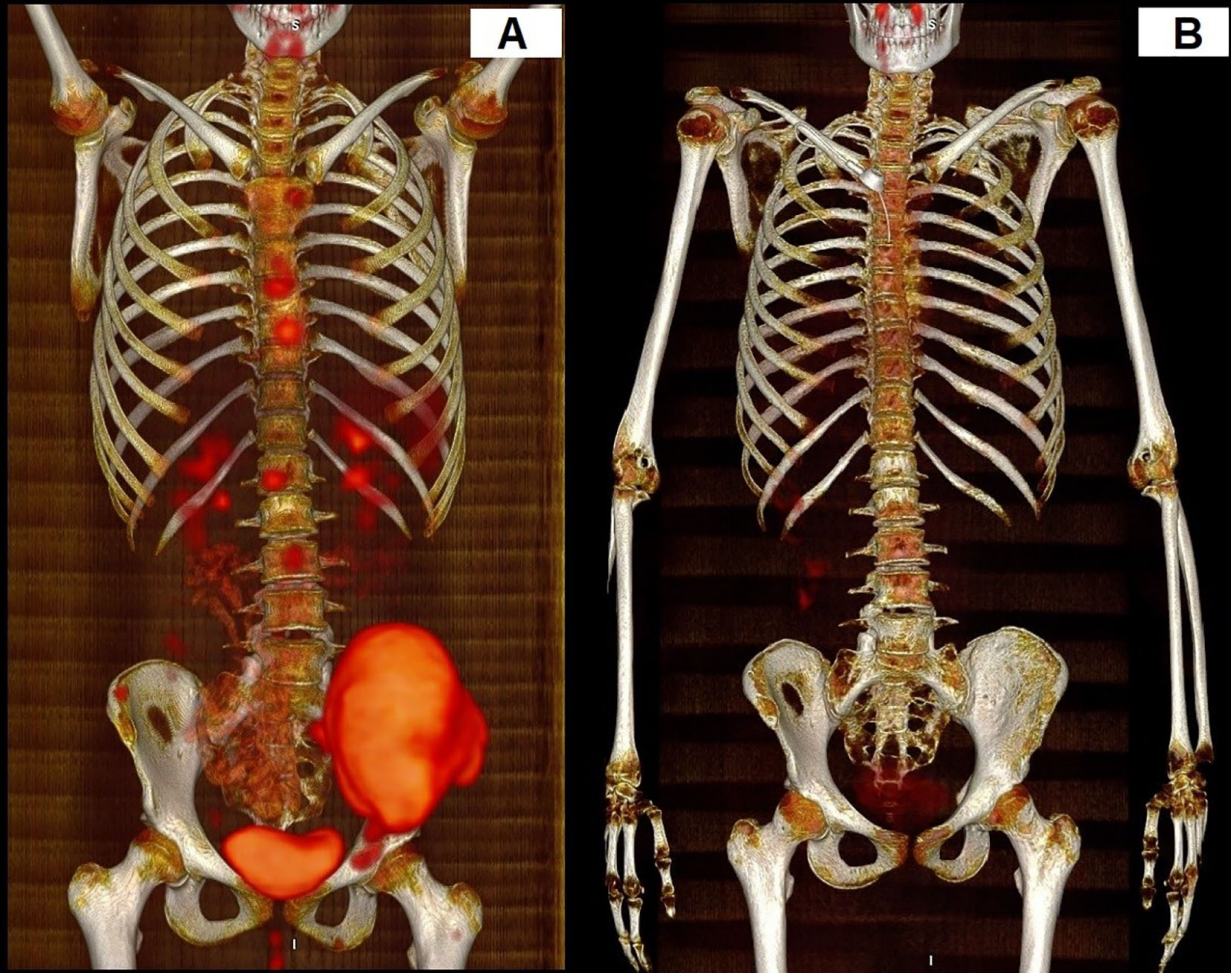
Response to the treatment in PET-CT. The patient with multisystem bone ES. (A) PET-CT before the treatment. (B) PET-CT after treatment.
